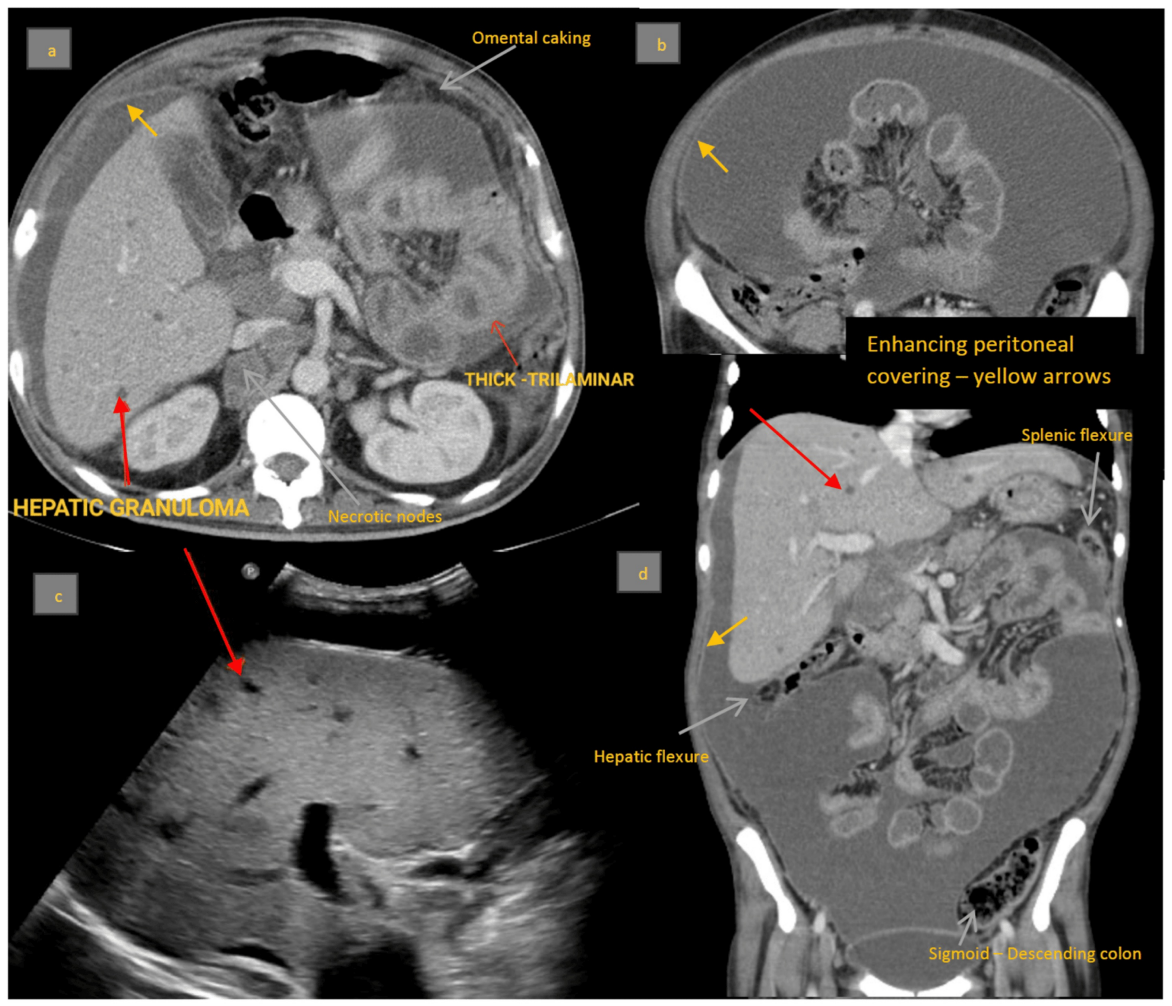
a: Axial post-contrast CT section showing hyperattenuating ascites with diffusely thickened, enhancing peritoneal covering, omental caking, and multiple enlarged and necrotic abdominal lymph nodes. b: Axial post-contrast CT section with central clumping of encapsulated small bowel loops without obstruction. c: Ultrasound of the abdomen showing multiple well-defined hypodense lesions in the liver, likely granulomatous infection. d: Coronal contrast CT section showing loculated ascites with enhancing peritoneal covering suggestive of abdominal cocoon (note the hepatic and splenic flexures and descending colon outside the cocoon). Overall imaging features: wet-type abdominal tuberculosis. CT: computed tomography
